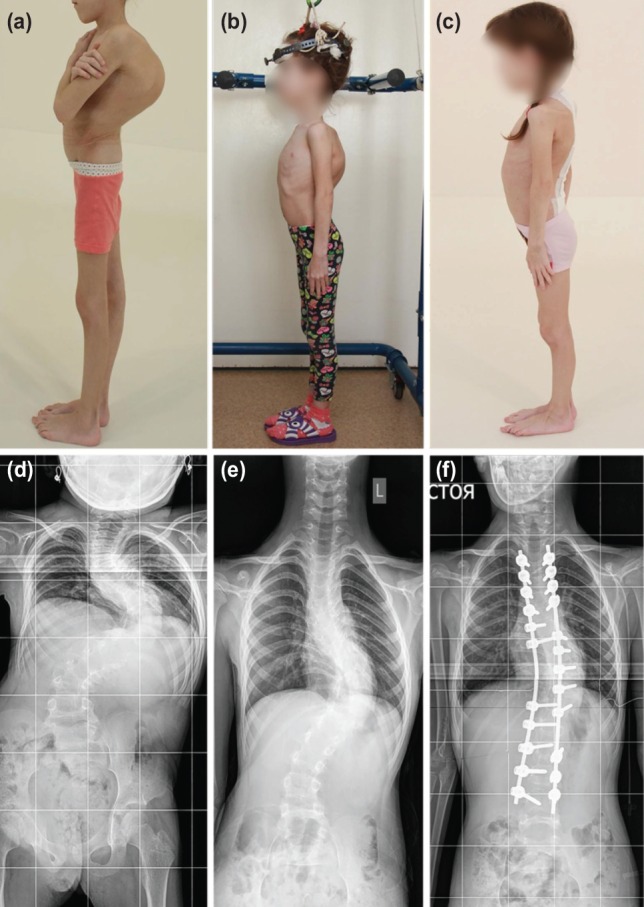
7-year old patient with undifferentiated connective-tissue dysplasia (Case 1). Images (a) pre-operative, (b) on traction and (c) post-operative. Radiographs (d) pre-operative, (e) on traction and (f) post-operative.
